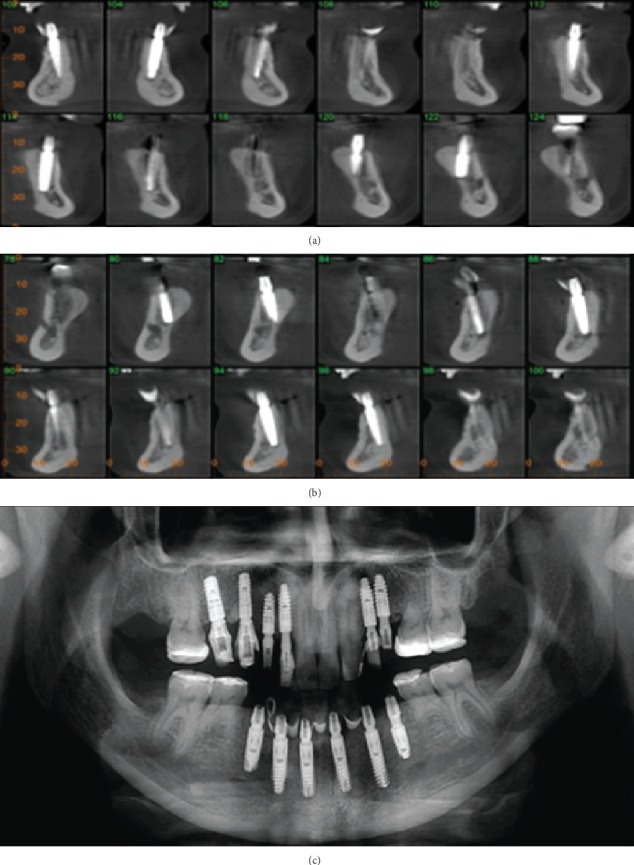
Four-year follow-up CT, showing the stability of the bone tissue around the implants (a, b). Panoramic radiograph of the 4-year follow-up (c).
